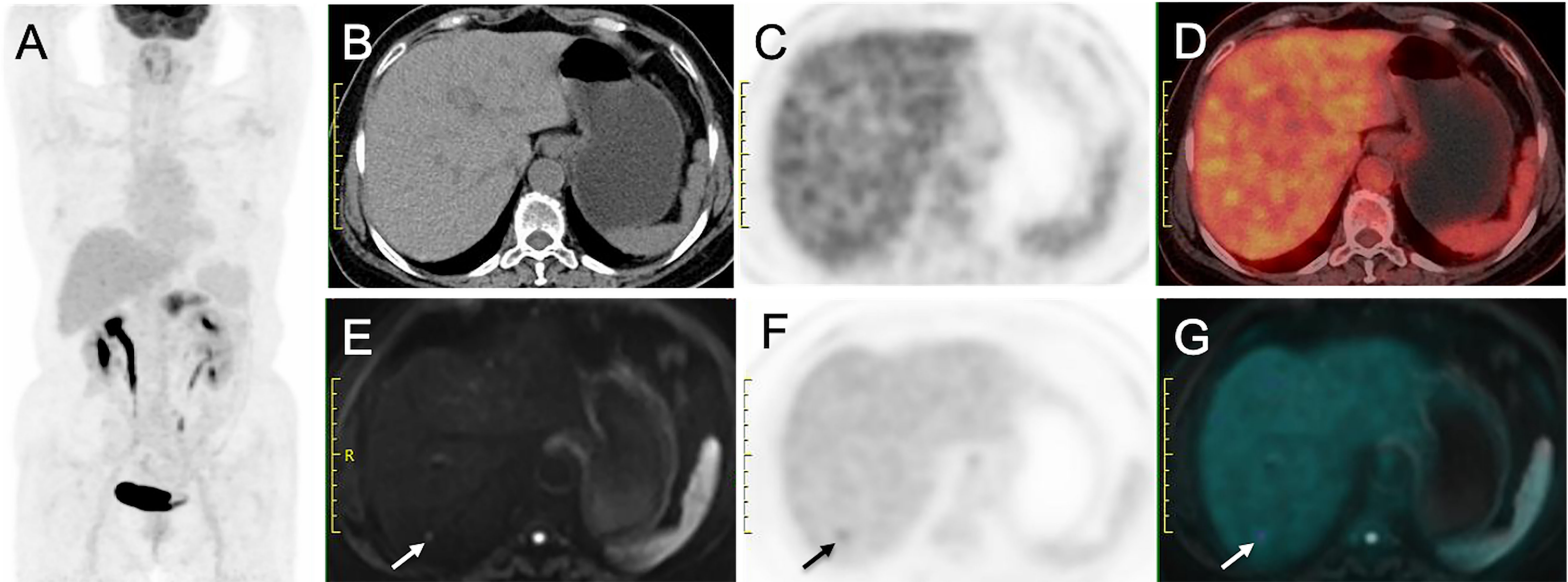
Liver metastasis detected on PET/MRI but not on PET/CT. (A) MIP from PET/CT showing increased uptake in pancreas. (B) Nonenhanced CT image, (C) Early PET image and (D) PET/CT fusion image show no hypoattenuating or hypermetabolic lesion in liver. (E) DW image (b = 800 sec/mm2) shows a nodule with restricted diffusion (arrow). (F) Delayed PET image shows a hypermetabolic lesion in liver. (G) Corresponding PET/MR imaging fusion image shows the nodule with both hyperintense and hypermetabolism. This patient was diagnosed as having stage M1 disease on PET/MR imaging but stage M0 disease on PET/CT.
